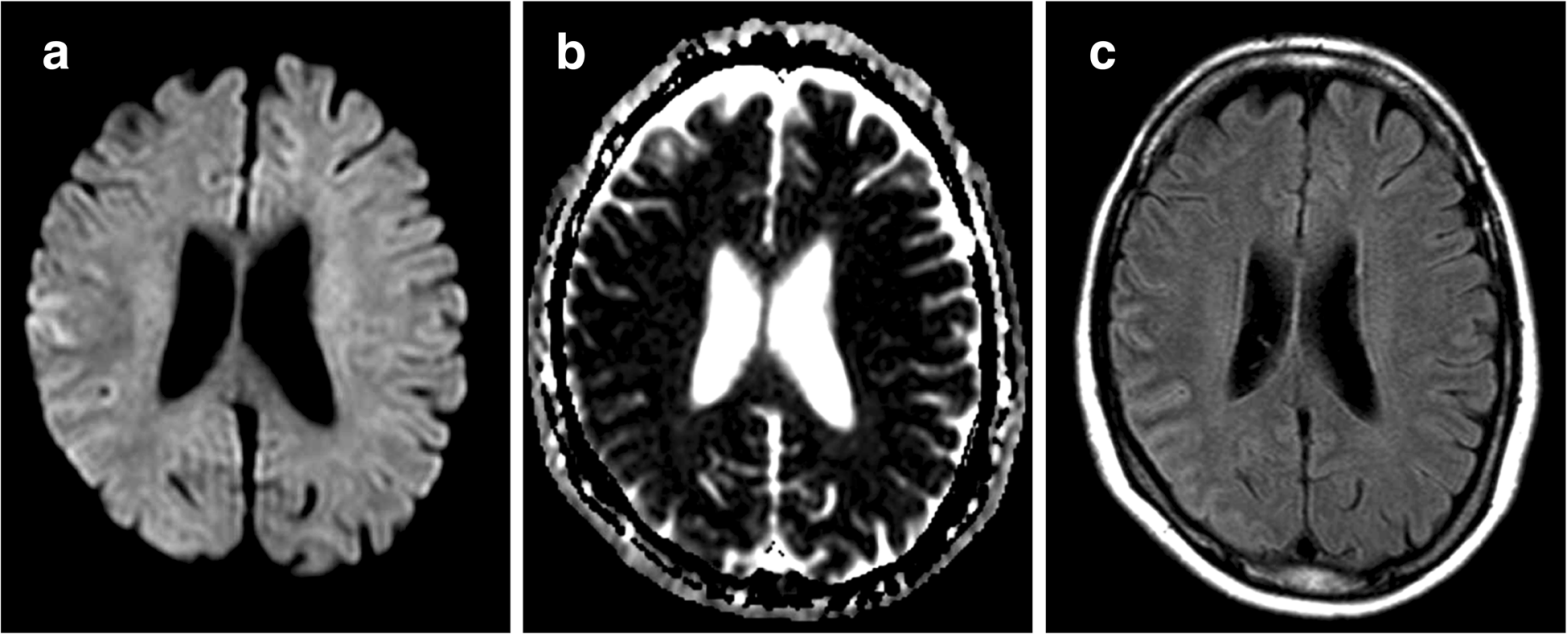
MRI findings at day 1. DWI (a) demonstrates a linear high-intensity lesion in the right frontotemporal cortex. Reduced ADC of this lesion is seen on the ADC map (b), and this cortical lesion appears as a high-intensity lesion on the FLAIR image (c). MRI = magnetic resonance imaging, DWI = diffusion-weighted imaging, ADC = apparent diffusion coefficient, FLAIR = fluid attenuated inversion recovery
